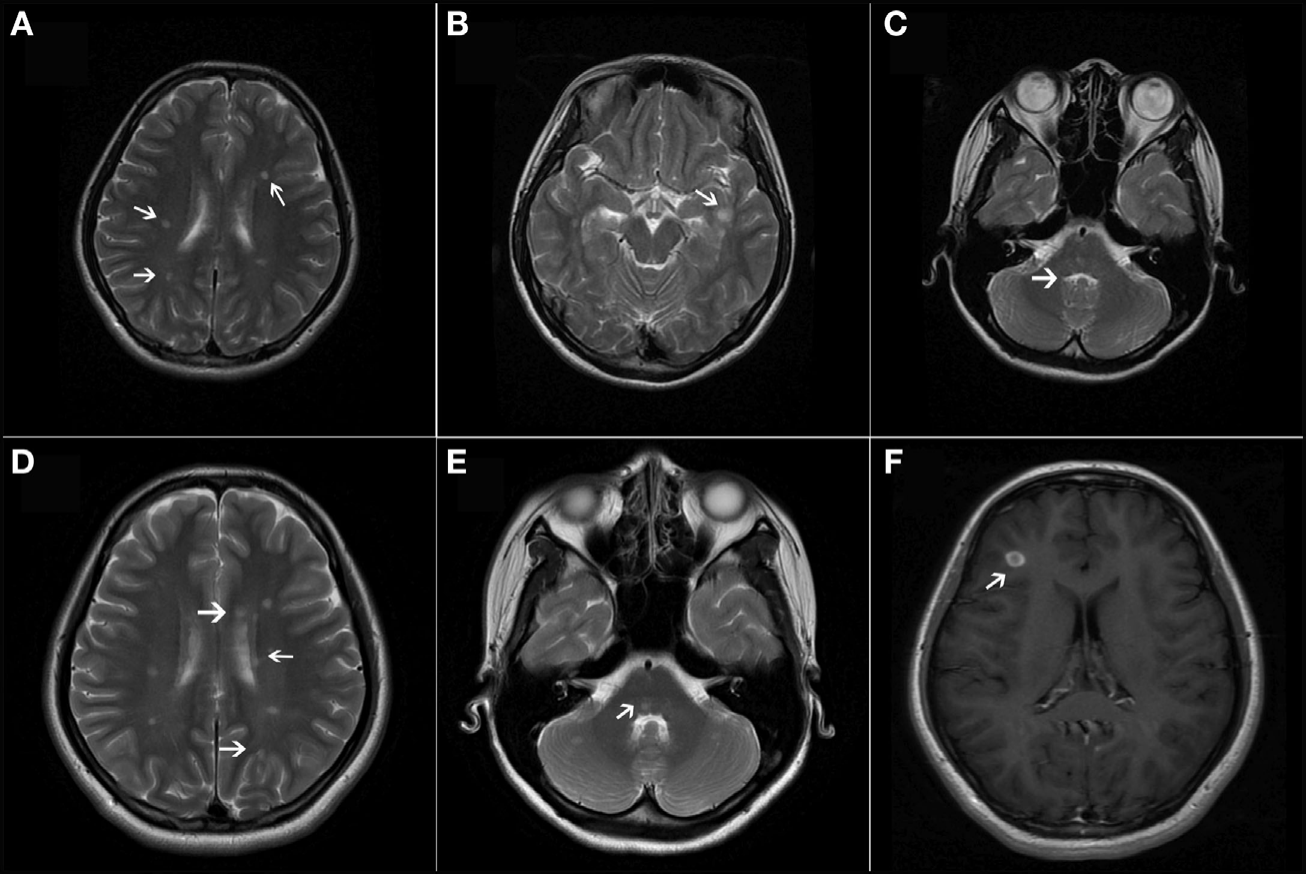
(A–C) T2-weighted axial magnetic resonance imaging (MRI) revealed multiple, ovoid-shaped, hyperintense lesions in the bilateral periventricular regions, the juxtacortical area of the left inferior temporal lobe, and the pons. (D,E) T2-weighted axial MRI showed new lesions in the periventricular regions and the cerebellum. (F) T1-weighted contrast-enhanced image showed one asymptomatic gadolinium-enhancing lesion in the right frontal lobe.
